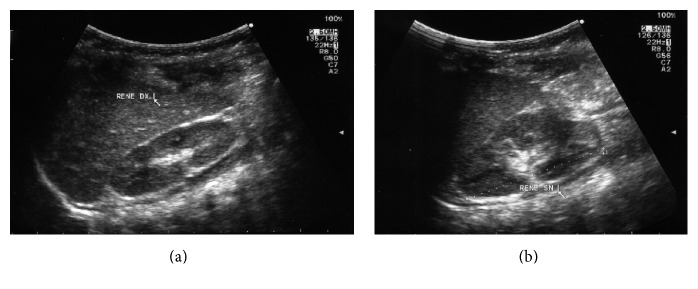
Renal ultrasound: absence of hydronephrosis was showed during a complete abdomen US examination; (a) right kidney and (b) left kidney.
